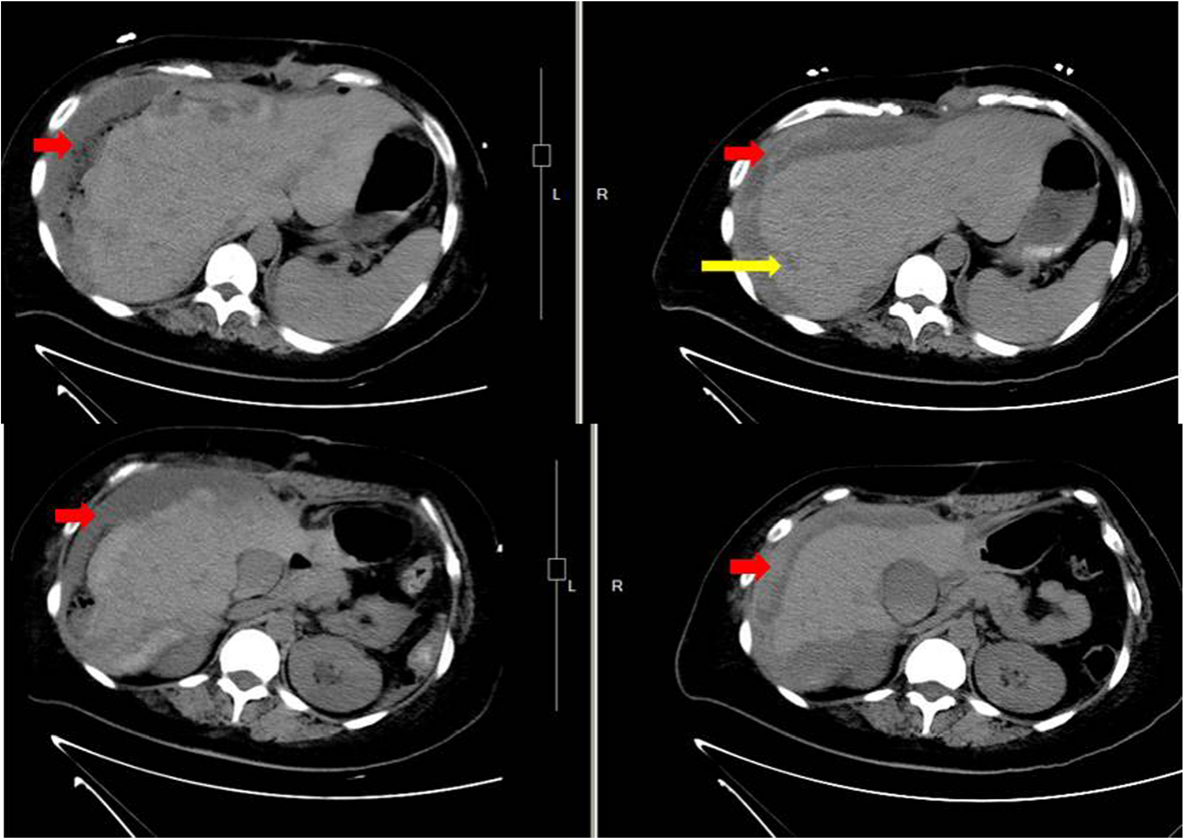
Abdominal CT scans on the 3rd day (left) and 13th day (right) post the second surgery. Red arrows (hypodense collection) show perihepatic effusion. Yellow arrow shows the ruptures of the liver
